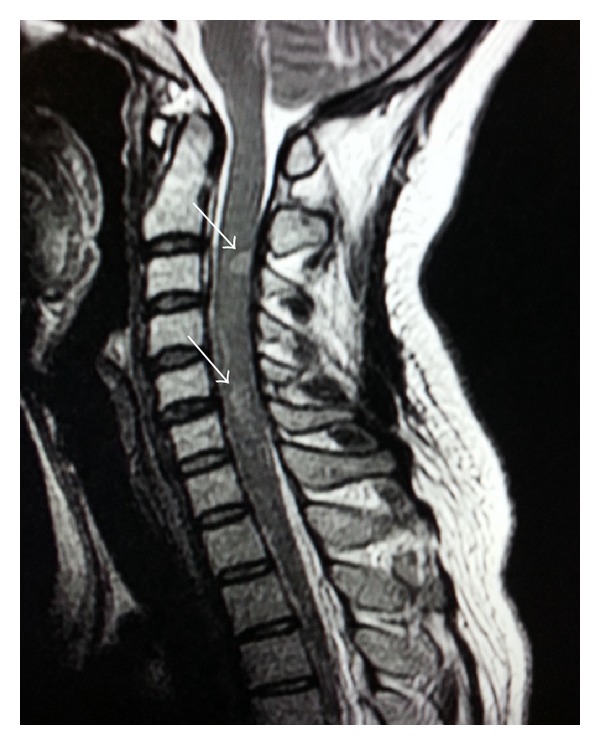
MRI cervicodorsal spine showing multiple plaques of abnormal signal on T2W image (arrows).
